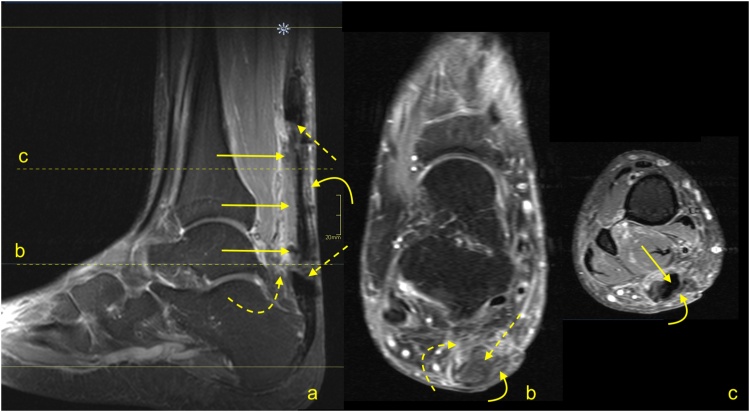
Status after Achilles tendon reconstruction 2 years ago. Asymptomatic patient, with good clinical function of the tendon. MRI (a–c) revealed the preserved tendon continuity with the native tendon (dashed arrow). The reconstructed tendon segment has a heterogeneous signal and an irregular outline (straight arrow), which are expected postoperative changes. A slightly higher signal of Kager's fat pad (curved dashed arrow) and the paratenon (curved arrow) are postoperative changes. A- T2- weighted with fat suppression, b and c- PD- weighted with fat suppression.
